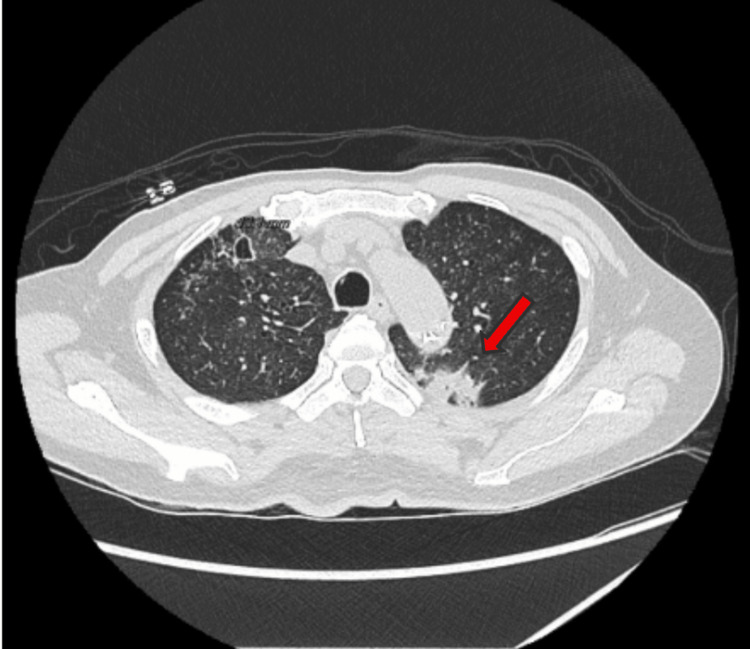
Progressive cavitary pulmonary consolidation abutting the descending thoracic aorta Axial CT of the chest at 12 weeks demonstrating a cavitary consolidative mass within the left lung adjacent to the descending thoracic aorta measuring 2.7×2.8×8.4 cm (red arrow). The lesion demonstrates evolving cavitary components compared with imaging obtained 12 weeks earlier, concerning for infection or malignancy.
